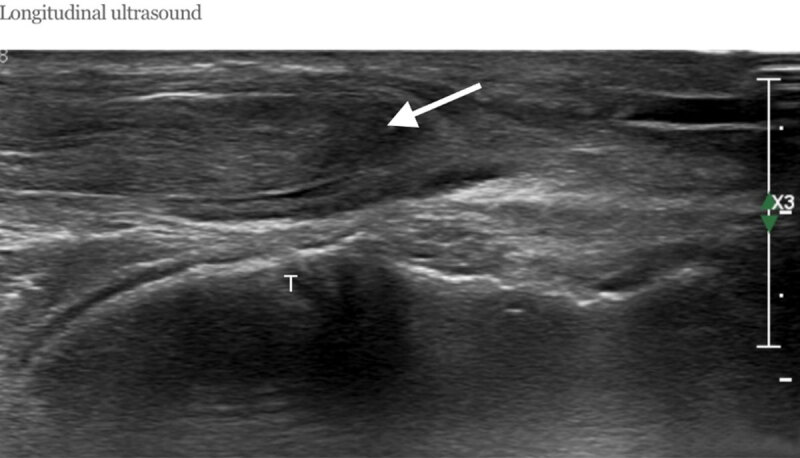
Magnetic resonance imaging (MRI) confirmed a full-thickness tear of the TAT with slight retraction of the tendon on sagittal fat-suppressed (FS) T2-weighted Image (WI) (Figure 2, arrow). Axial FS T2-WI showed a swollen heterogeneous TAT above the ankle joint (Figure 3A, arrow) and absence of the TAT below the tibiotalar joint (Figure 3B, arrow).
